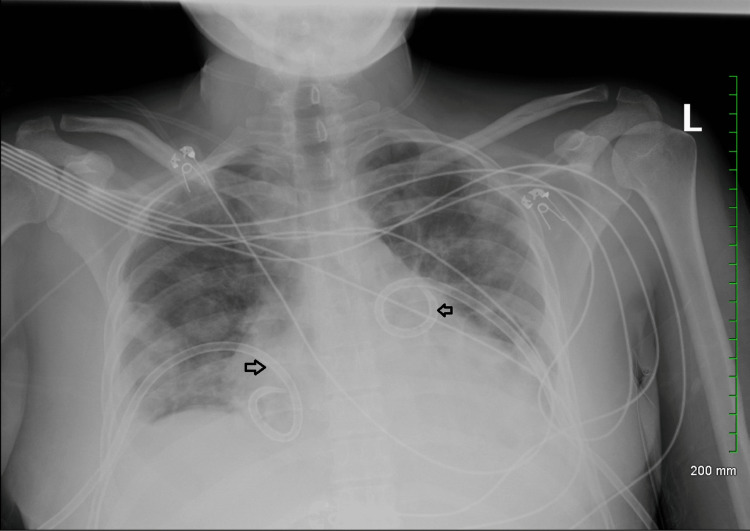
Chest X-ray showing improvement in bilateral pneumothorax after insertion of bilateral chest tubes. Bilateral chest tubes labeled with transparent arrows.
